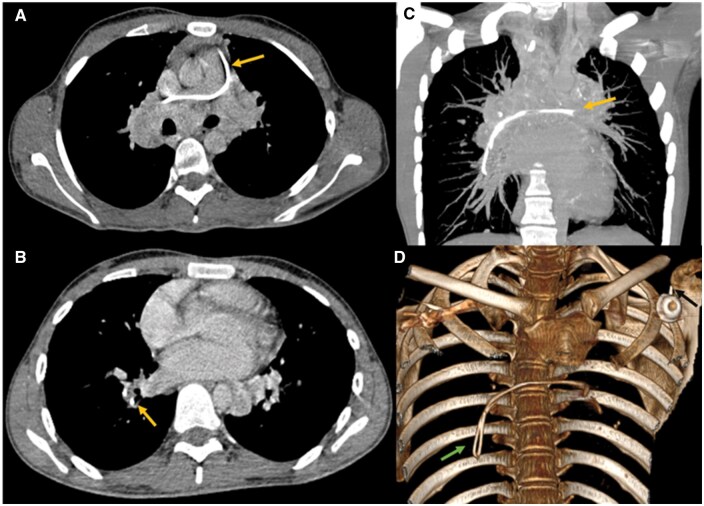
(A) Axial chest CT scan mediastinal window showing the fragmented catheter in the pulmonary trunk extending to the right pulmonary artery. (B) Axial chest CT scan mediastinal window showing, the fragmented catheter the inferior right lobar artery (arrow). (C) Chest CT scan with maximum intensity projection reconstruction in coronal view showing the fragmented catheter extending from the pulmonary trunk to the inferior right lobar artery (arrow). (D) 3D reconstruction showing the external chamber (black arrow) and the fragmented catheter (green arrow).
